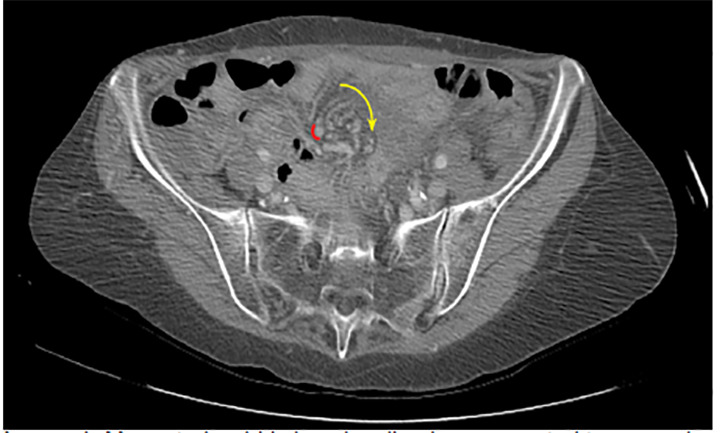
Mesenteric whirl sign visualized on computed tomography (yellow arrow).
